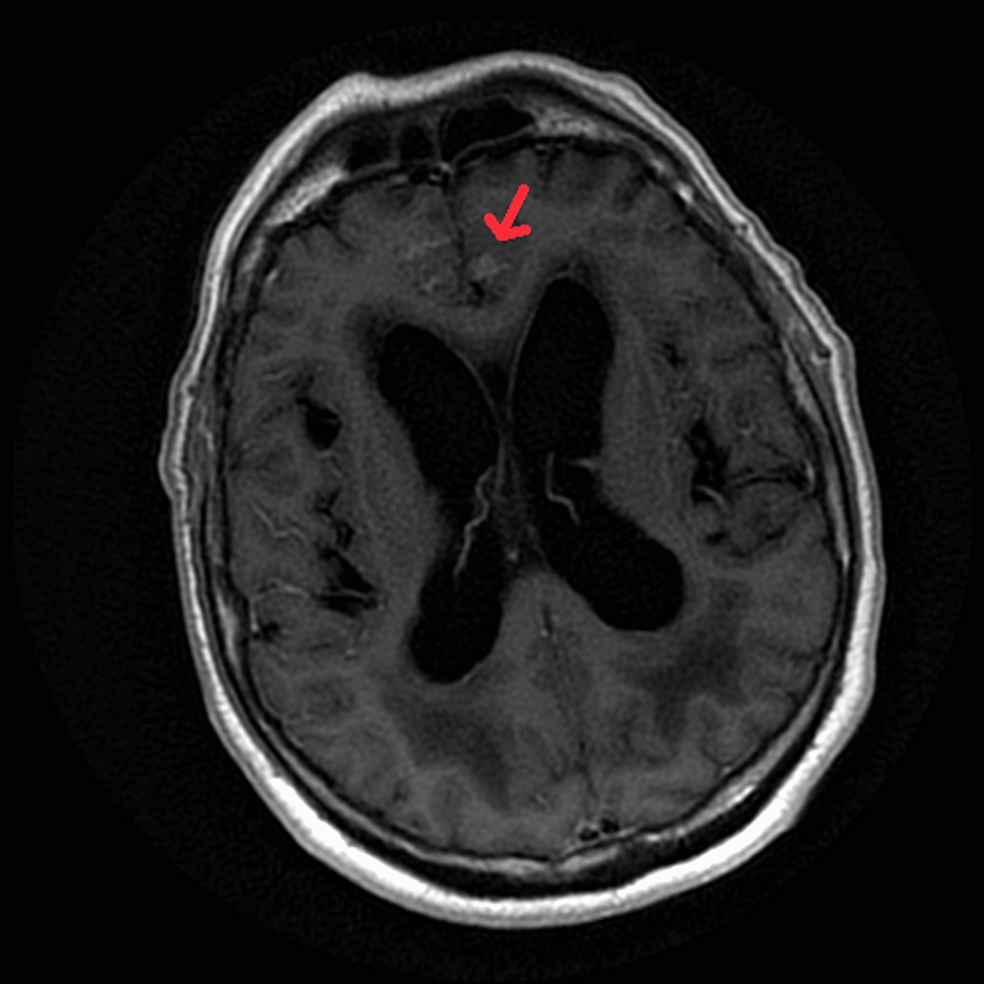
MRI of the brain with and without contrast shows meningeal enhancement within the sulci of the left frontal lobe.
